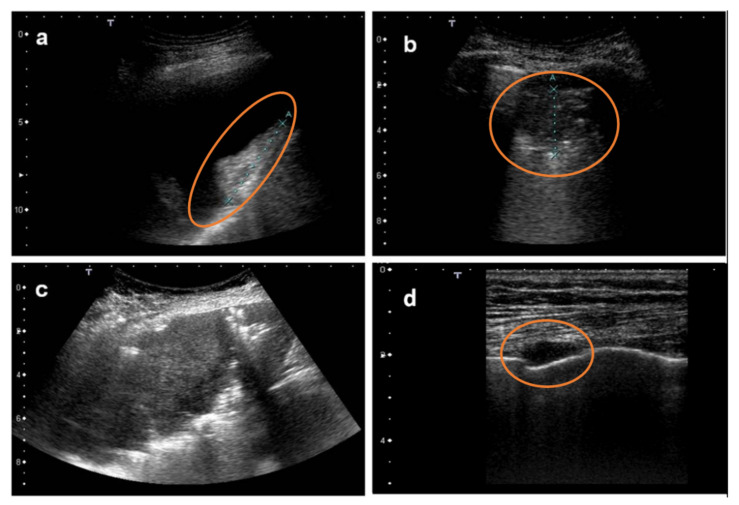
Image (a) shows an ultrasound scan of a patient with large pleural effusion and a coarse vegetation on the diaphragmatic surface (metastatic carcinoma in red circle). Image (b) shows lung consolidation with poor ventilation (pneumonia in red circle). Image (c) shows a large lung consolidation (lung cancer). Image (d) shows pleural neoplasia (mesothelioma in red circle).
